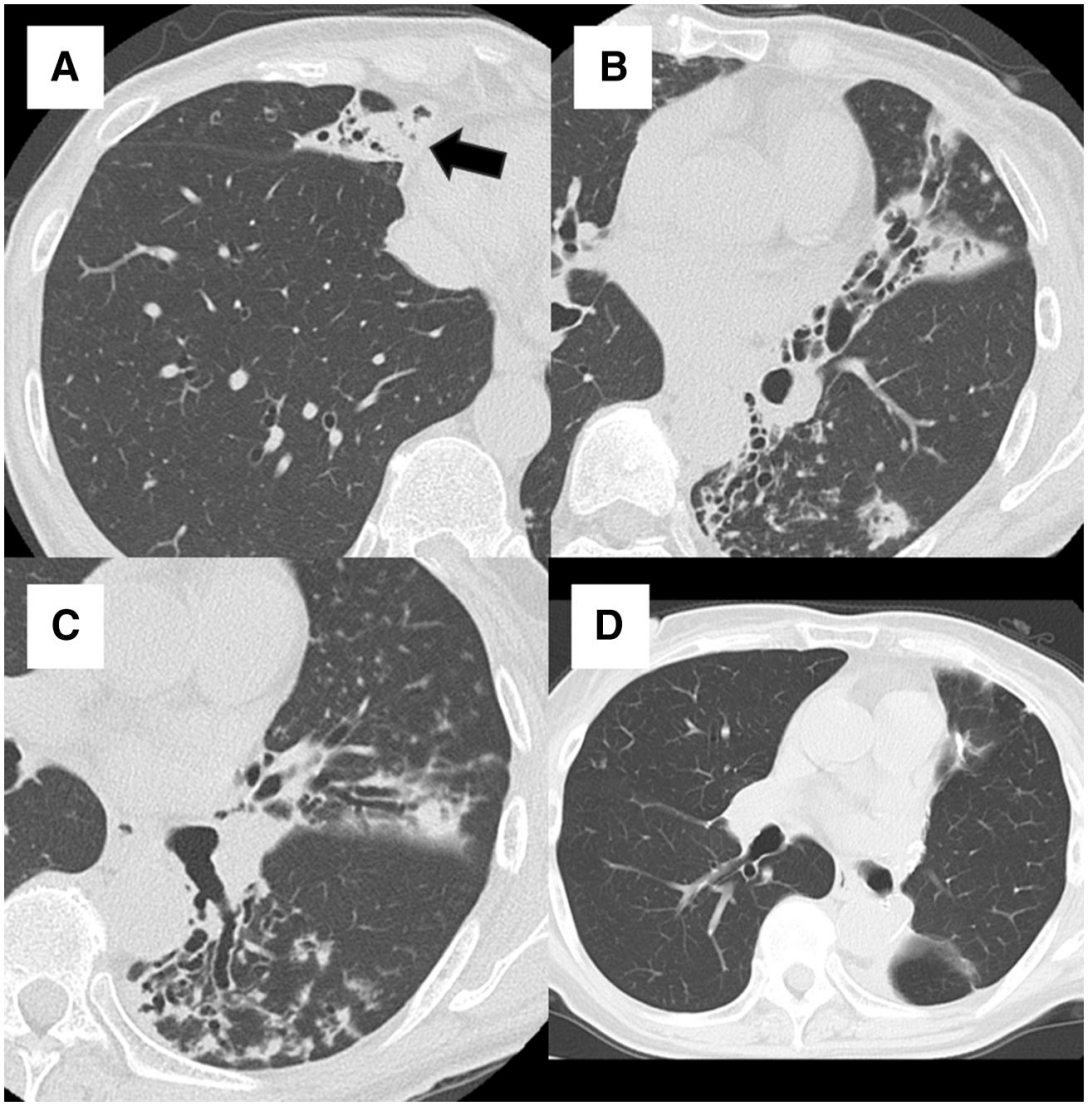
A Case of MR-MAC Pulmonary Disease. CT images of a 68-year-old woman with MR-MAC lung disease. (A-C) Preoperative axial views show destructive lesions in the right middle lobe, left lingula, and left lower lobe. Bilateral anatomical lung resections were performed in 2 stages. (D) Postoperative CT shows no residual lesions. The patient remains relapse-free and off antimicrobial therapy. Abbreviations: CT, computed tomography; MR-MAC, macrolide-resistant *Mycobacterium avium* complex.
